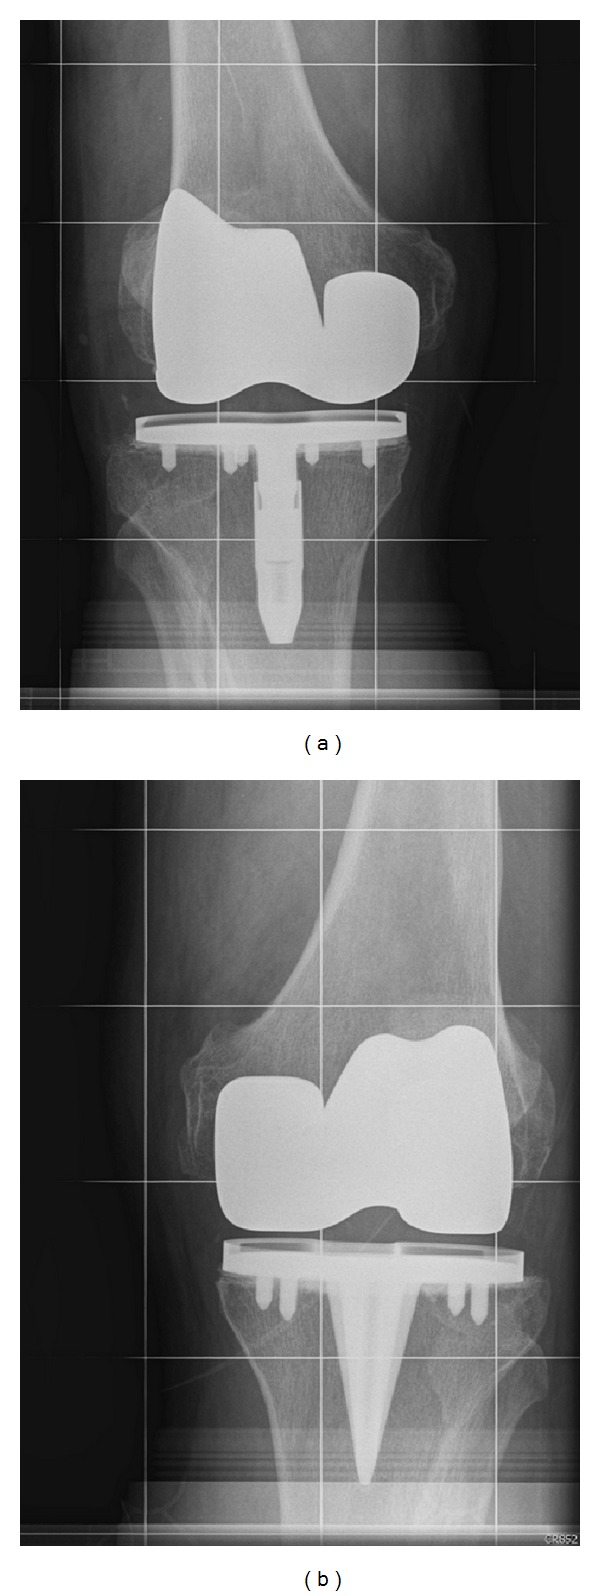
Anteroposterior radiograph of a bilateral TKA patient implanted with (a) the Profix Total Knee System (right knee) and (b) Natural Knee II (left knee) used in the historical control study.
